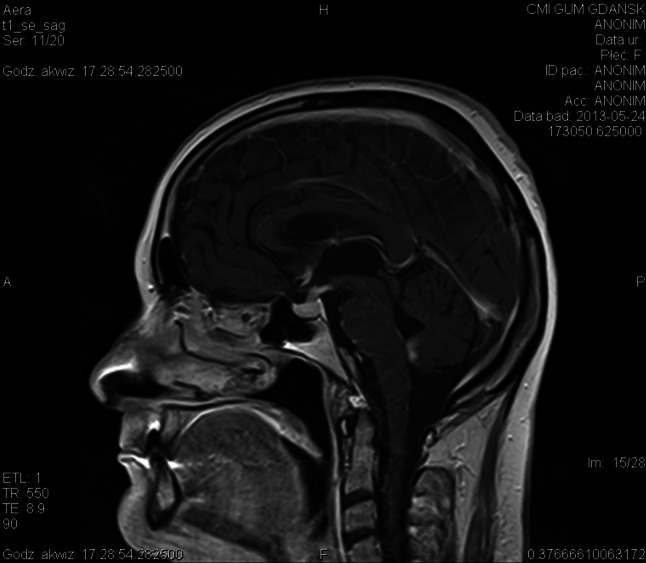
Neurosarcoidosis in MRI brain in sagital plane: T1-weighted contrast-enhanced MR image shows basal leptomeningeal enhancement and an extensive enhancement of the pituitary gland and stalk, which is markedly enlargedGal67 scintigraphy—in 2/3 of patients changes can be revealed, however, in the majority they are unspecific; one characteristic image in scintigraphy, but also in FDG-PET, observed in sarcoidosis of the parotid and lacrimal glands is called a “panda sign” [45].FDG-PET—allows for a localization of changes in whole body and finding a characteristic intrathoracic pattern and/or “panda sign” may be highly suggestive for sarcoidosis; isolated NS needs additional diagnostic tests, because FDG-PET does not distinguish the changes in the course of malignancy or TB from NS [16, 40–47]; recently, it was revealed that a combination multiple fluorodeoxyglucose PET-avid lymph nodes with mild flurothymidine (FLT) PET uptake can be helpful in differentiating granulomatous inflammatory diseases such neurosarcoidosis from malignancy [42]; FDG-PET and FLT-PET are more useful methods in localizing the optimum site for a biopsy than Gal67 scintigraphy and MRI [37, 42].
